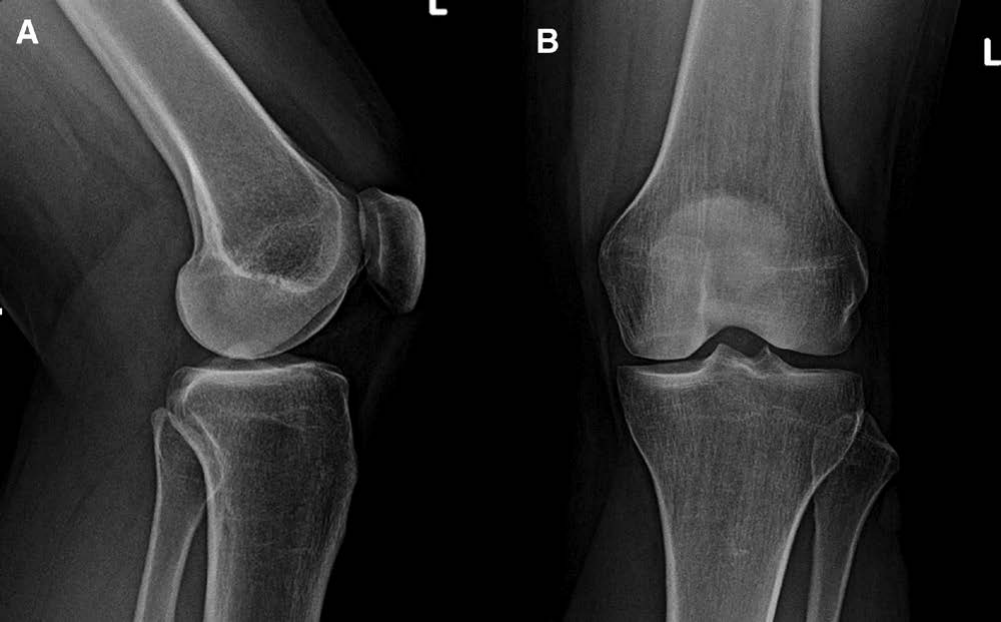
Lateral (A) and anteroposterior (B) knee radiographs showing no traumatic changes.
